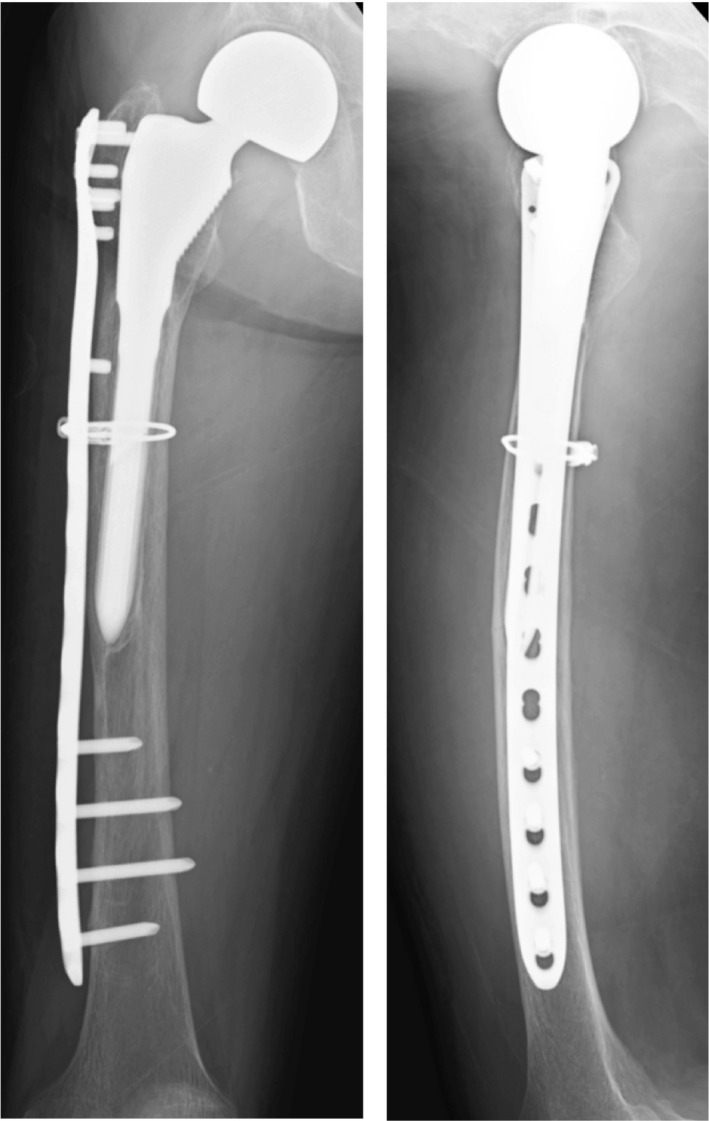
Radiographs of the right femur (anteroposterior and lateral) obtained 1 mo following preventive open reduction and internal fixation were performed
